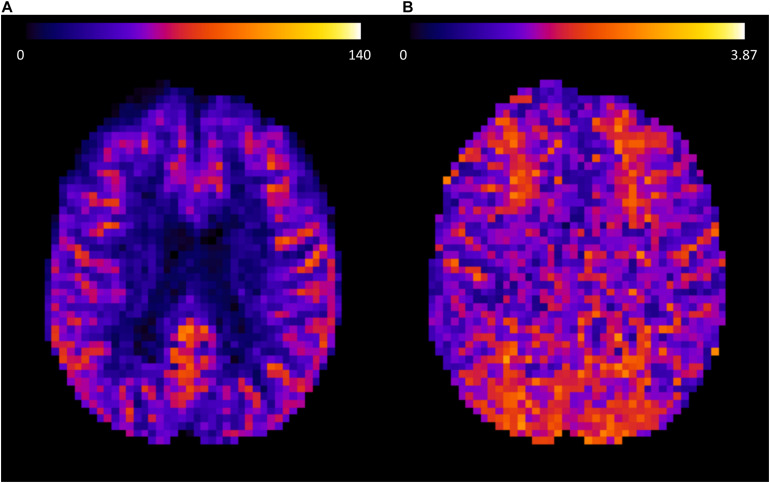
Axial slice of the cerebral blood flow (CBF) (A) and the arterial transit time (ATT) (B) result of a representative participant obtained from a pCASL acquisition at the Skyra MRI system. To visualize gray and white matter values within the same image, estimated results before a partial volume correction was applied are shown.
